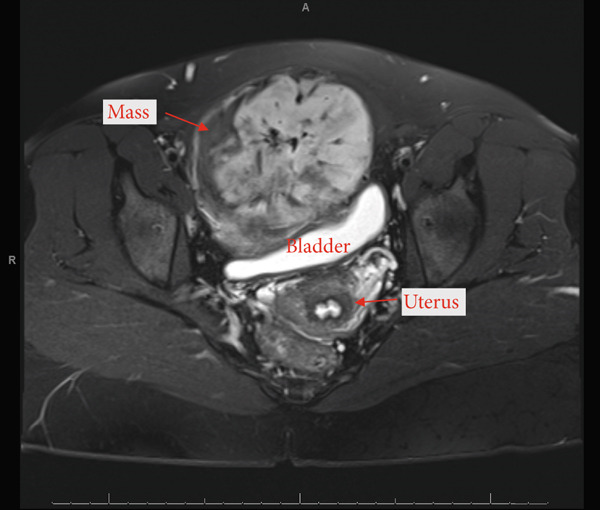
MRI pelvis demonstrating the mass to be distinct from the bladder and uterus.
